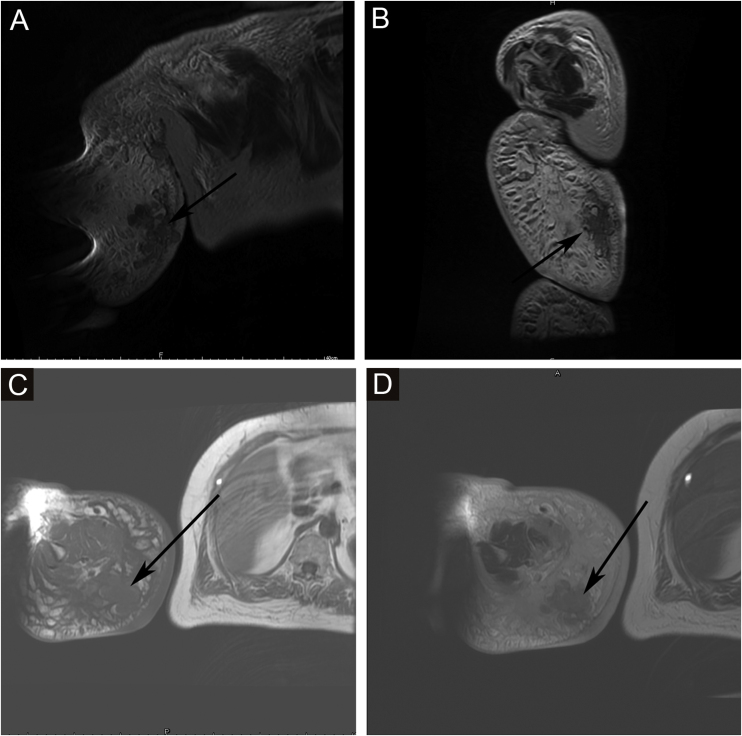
MRI showed nodules distributed on the surface of the skin, subcutaneous tissue and some muscles; it appeared slightly long T1 and short T2 signals. (A) AX fs T2 FSE. (B) AX fs T2 FSE. (C) AXI SE T1. (D) AX T2 FSE.
